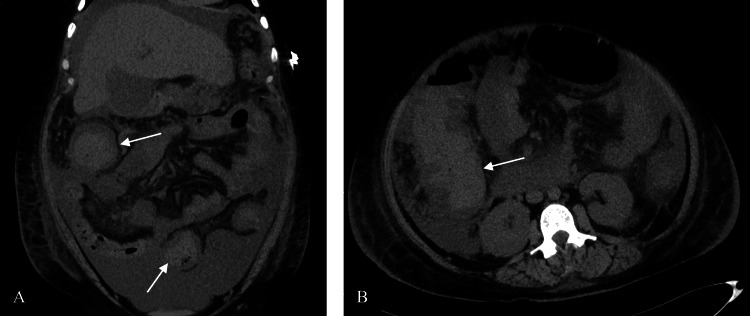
Pre-procedure computed tomography examination. Coronal (A) and axial (B) images from a computed tomography CT scan of the abdomen and pelvis without contrast reveal hyperdense intraluminal contents (arrows) throughout the colon, compatible with blood products.
